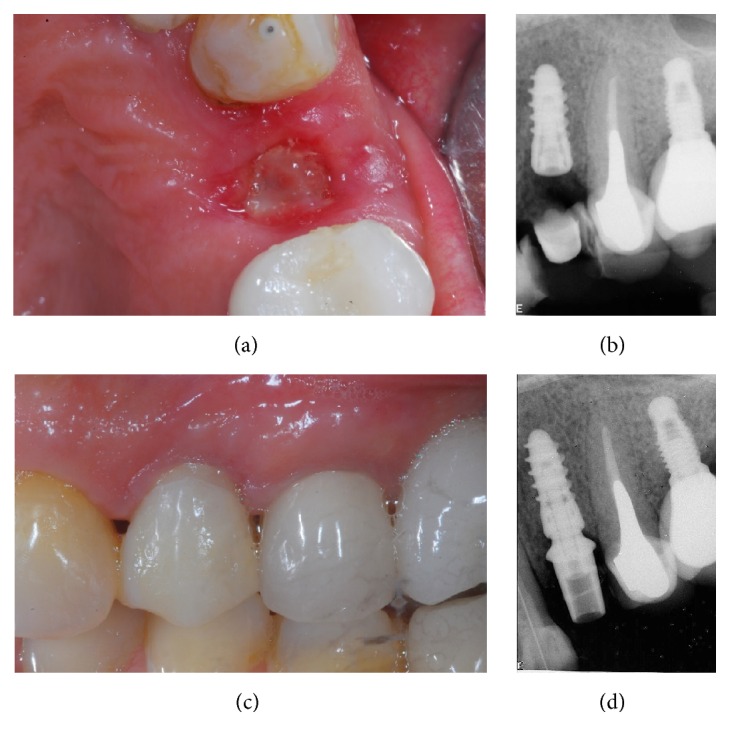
A single 3DP/AM titanium dental implant in a postextraction socket of the posterior maxilla: healing phases. (a) Ten days after surgery, sutures are removed. (b) Periapical rx 10 days after implant placement. (c) Three months later, a provisional restoration is placed. (d) Periapical rx at placement of the provisional restoration.
